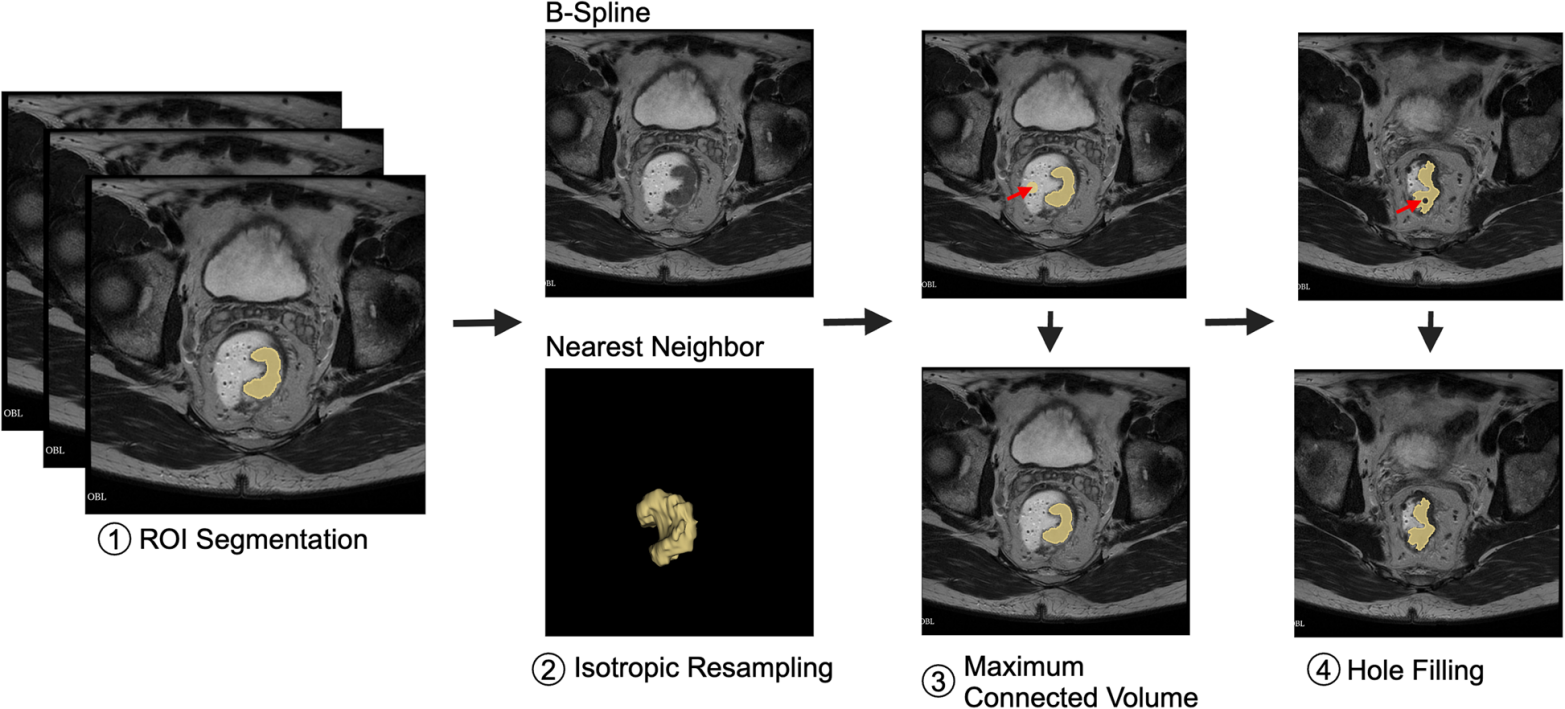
Overview of image pre-processing steps for data harmonization, including (1) ROI segmentation (yellow) delineated by data scientist and reviewed by radiologist, (2) isotropic resampling of MR images to a uniform spacing of 1 mm in the x, y, and z planes using B-Spline interpolation to preserve anatomical details, while segmentations were resampled using Nearest Neighbor (NN) interpolation to maintain boundary precision, balancing anatomical accuracy with segmentation fidelity, (3) extraction of the maximum connected component to remove stray pixels (denoted by red arrow), and (4) hole filling. Created in BioRender. Selby, H. (2025) https://BioRender.com/e41a602
